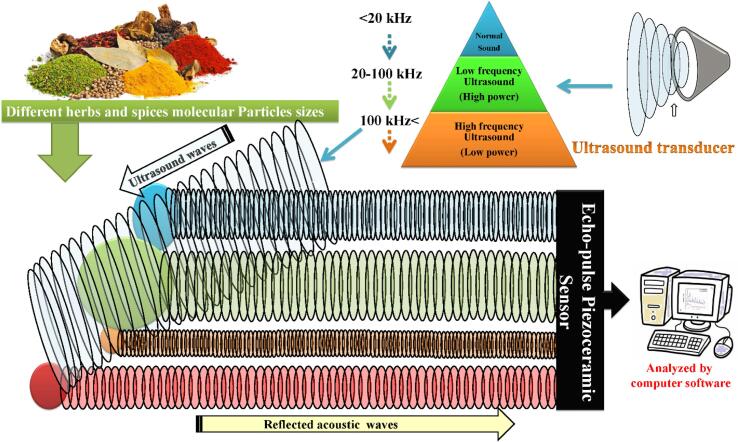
Principle effects of high-frequency and low-intensity ultrasound in measuring particle size of molecules.
